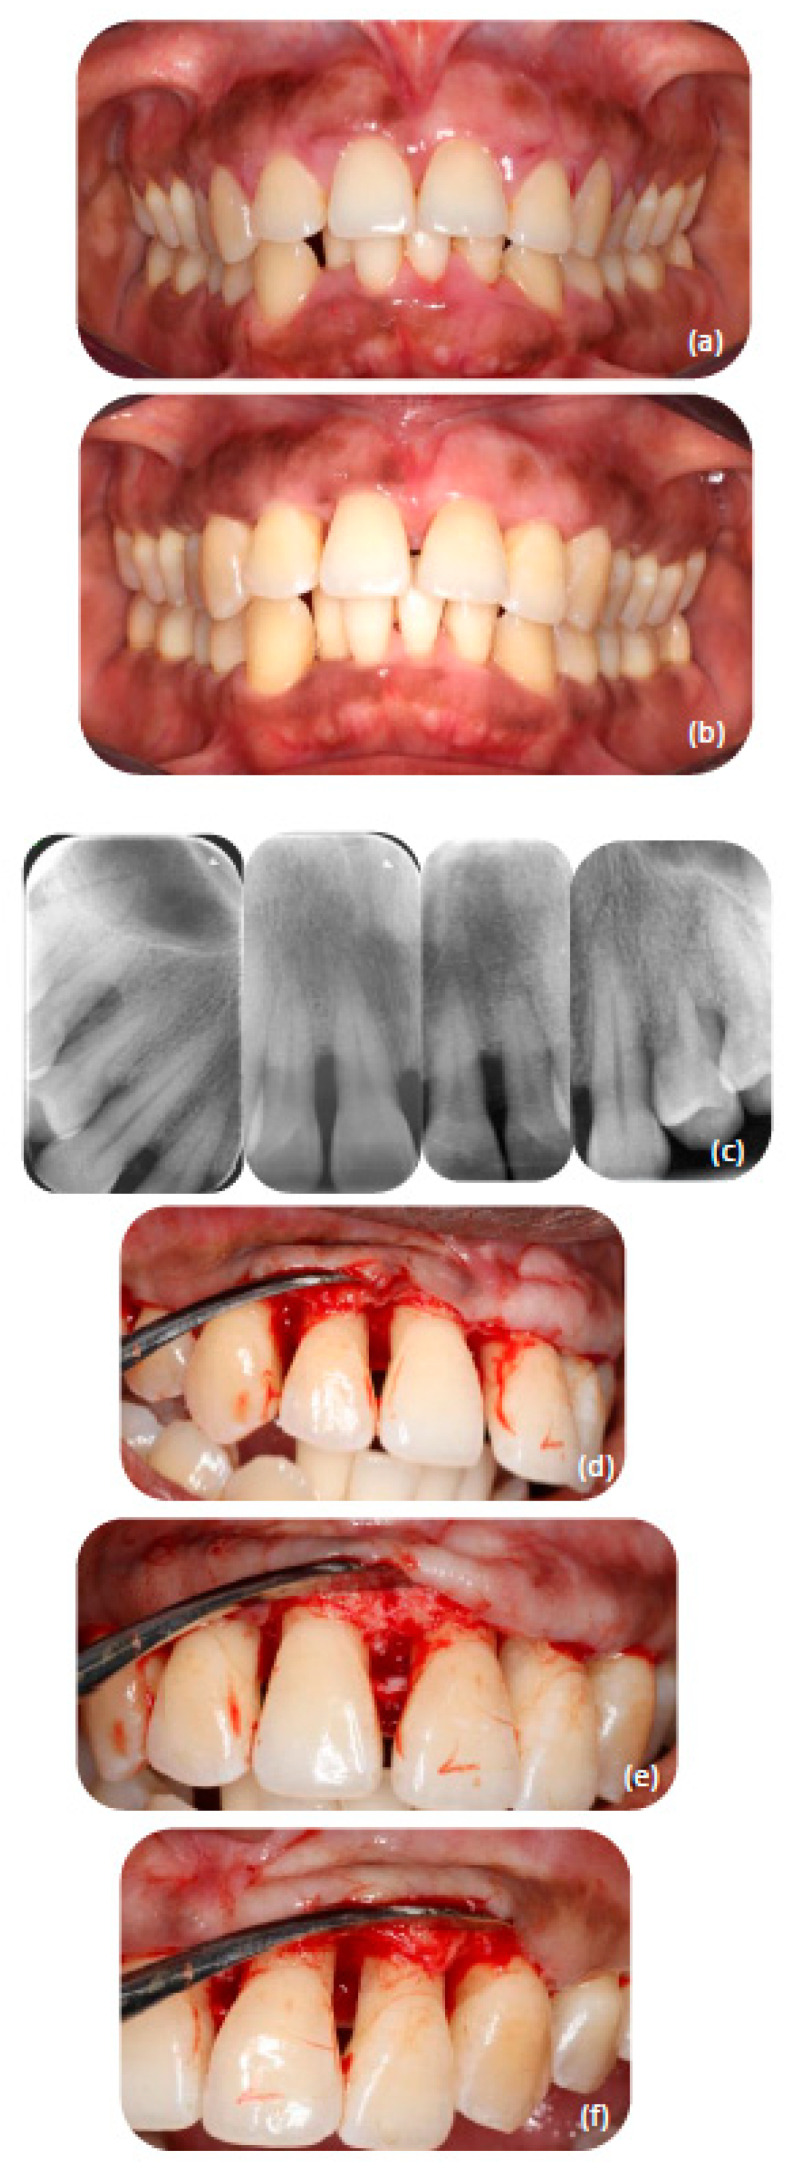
(a) Intraoral view at intake. (b) Intraoral view at 6 months after initial non-surgical treatment. (c) Radiographic overview of the maxillary anterior area. (d–f) Intraoral view during surgery.
